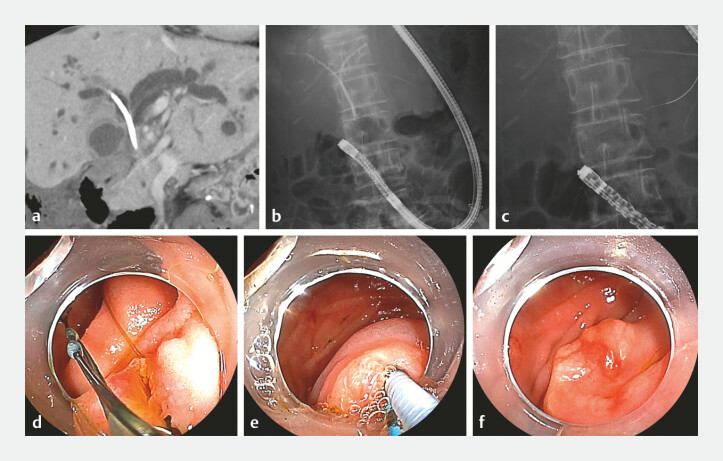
Preoperative computed tomography (CT) and balloon-assisted endoscopy (BAE) images.
